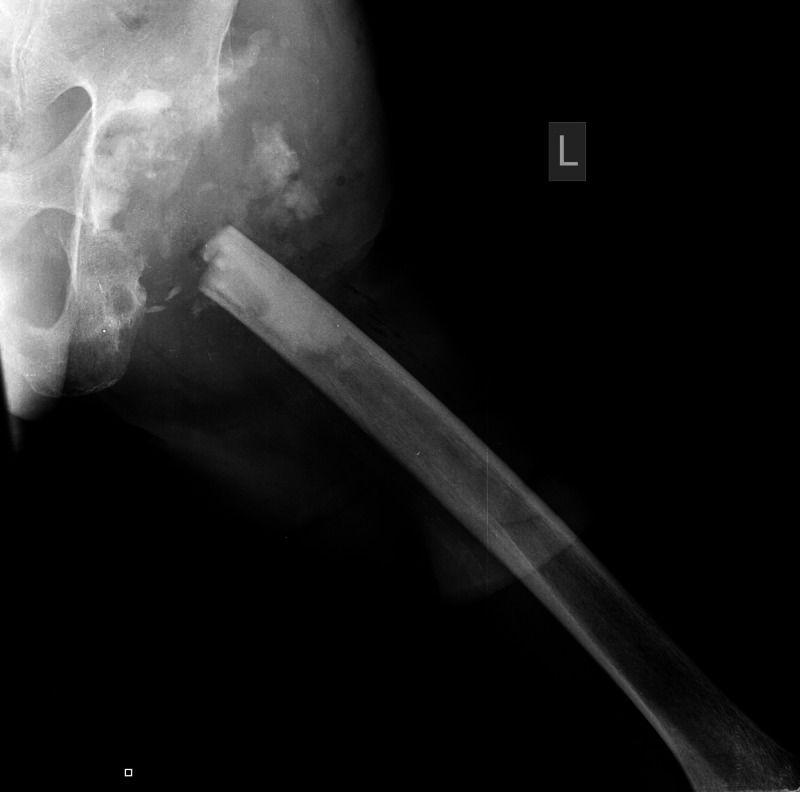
Postoperative anteroposterior left hip radiograph showing the radiologic outcome of the last procedure where the proximal femur was osteotomized to safe margins
